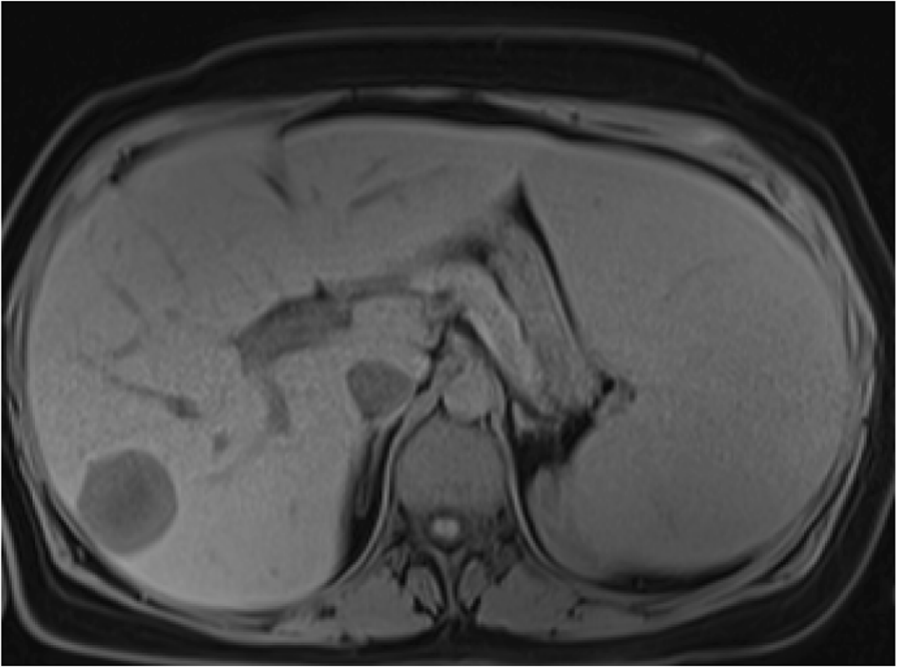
An abdominal magnetic resonance imaging scan showing a nodule in liver segment VII, hypointense on T1, measuring 37 mm × 32 mm
